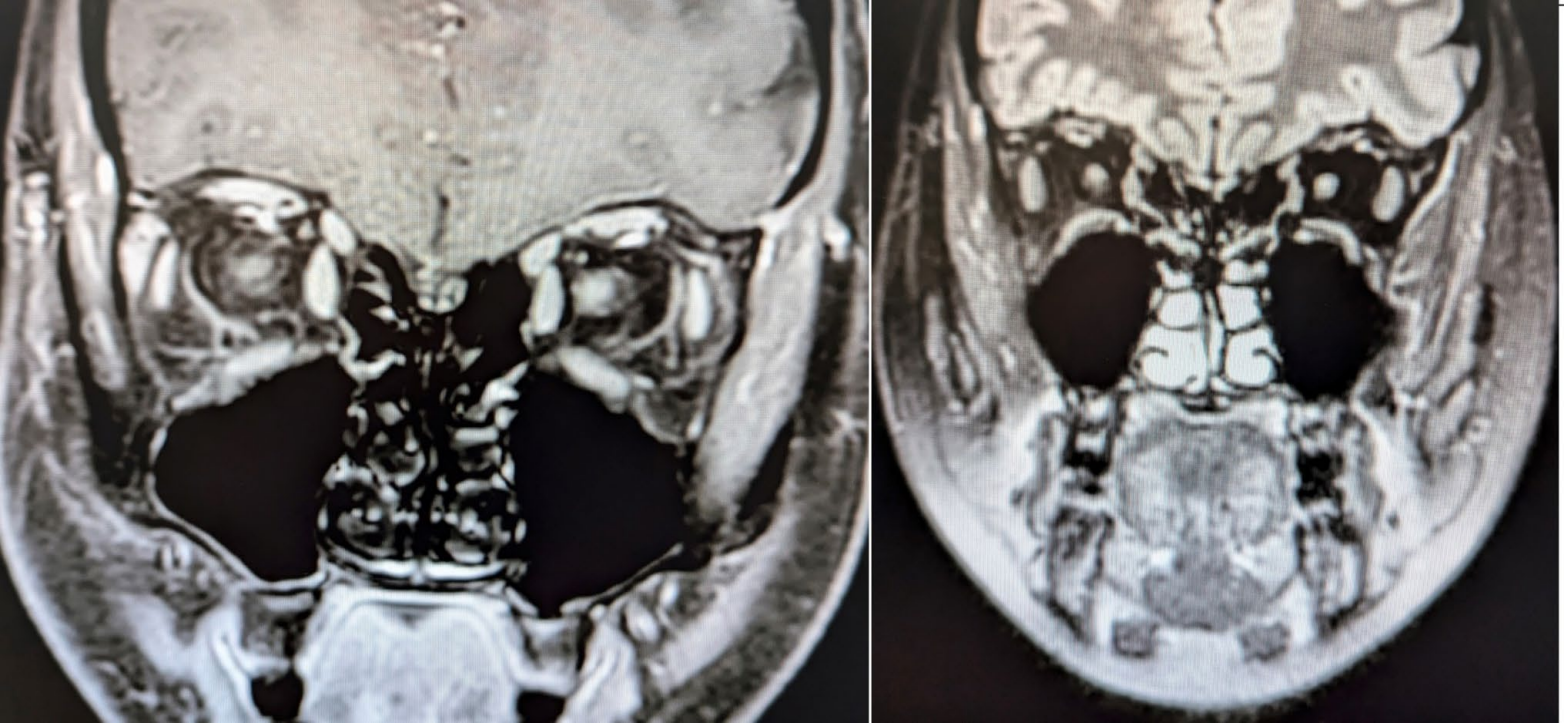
Optic nerve lesion shown on orbital MRI (coronal T1-weighted sequence after gadolinium contrast injection and coronal T2 weighted sequence)
